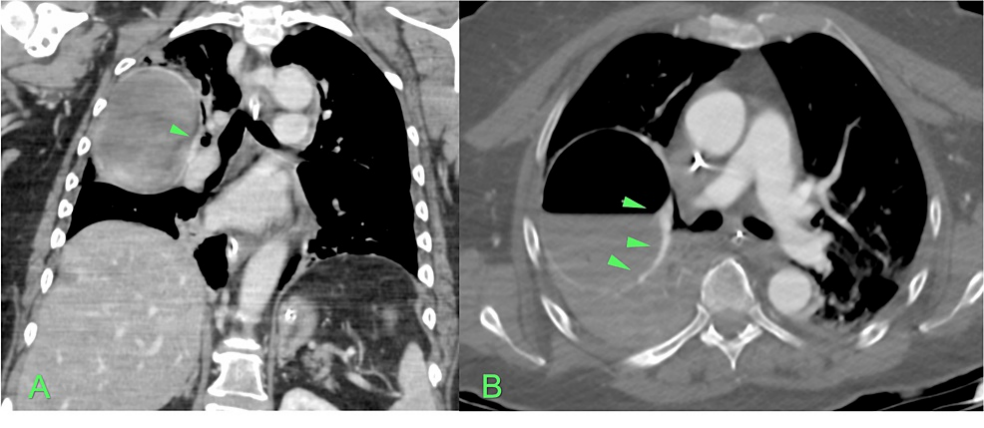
Thoracic CTA with coronal (A) and axial (B) cut of the bulla surrounded by pulmonary venous vascular structures (green arrows)
